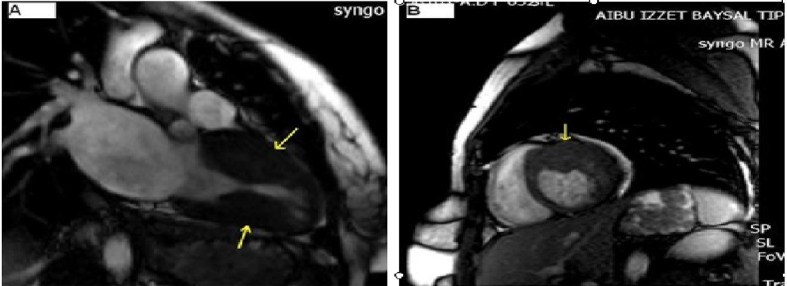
Cardiac MRI imaging. Asymetric hypertrophy of the left ventricle posterior free wall and mid ventricular septum (A) and posterior-lateral free wall (B).
